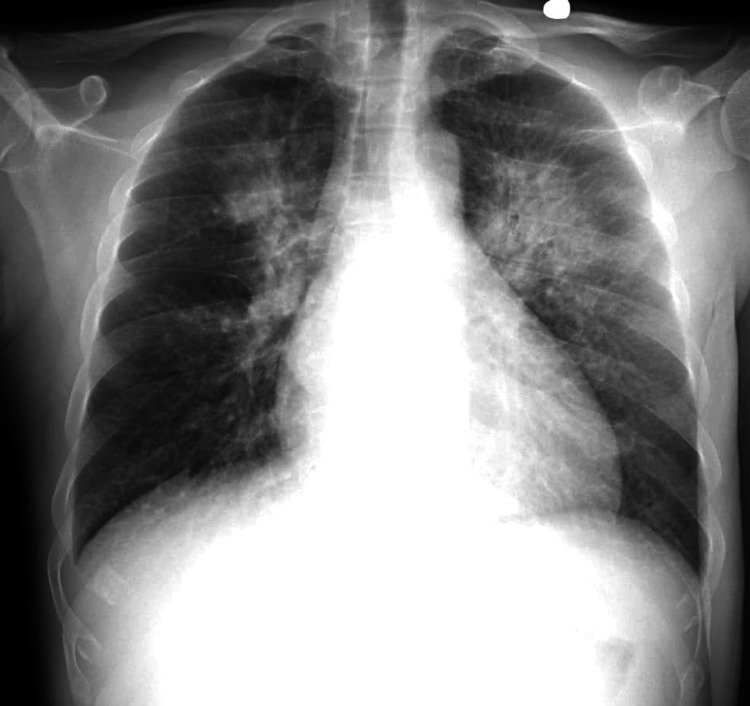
Chest X-ray showing diffuse bilateral infiltrates.
